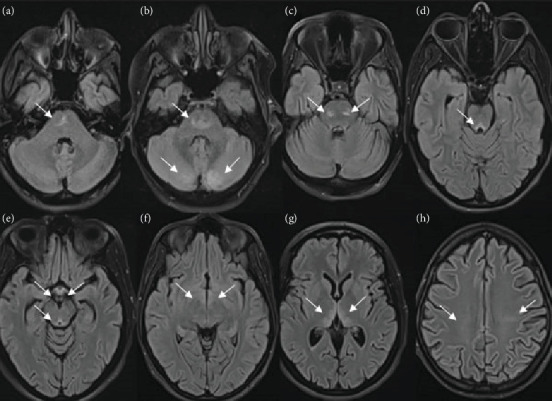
MRI of patients showing hypersignal lesions (arrows) on axial FLAIR in (a) the brainstem; (b) central pons and cerebellum; (c) red nuclei; (d) tectal plate; (e) mammillary bodies and periaqueductal region; (f) wall of the third ventricle; (g) thalami, paraventricular region, and frontal lobe; and (h) centrum semiovale.
